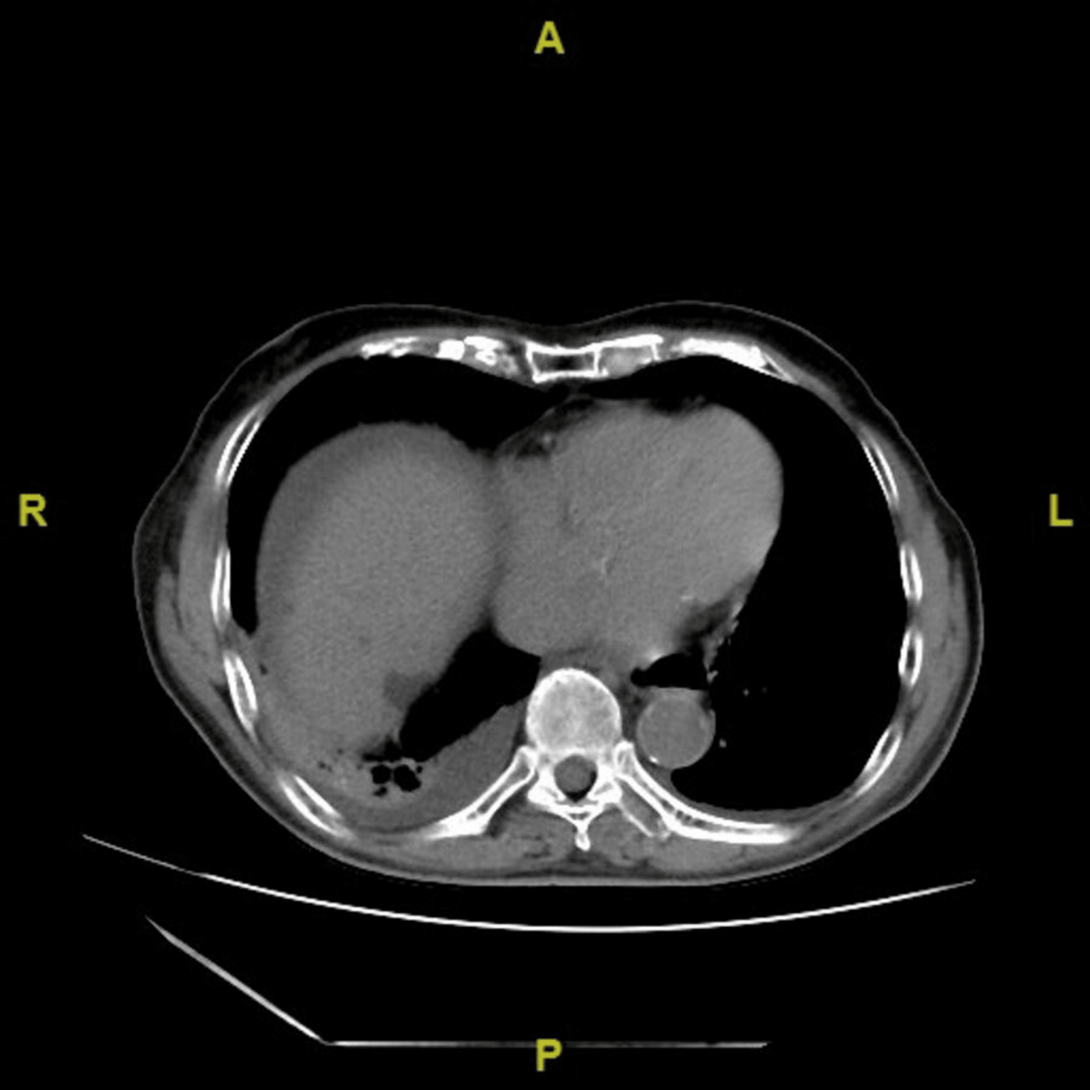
CT scan showed right lower lobe atelectasis and pleural effusion Non-contrast CT on admission showing right lower lobe atelectasis and pleural effusion. These findings contributed to the patient’s increased perioperative risk.
